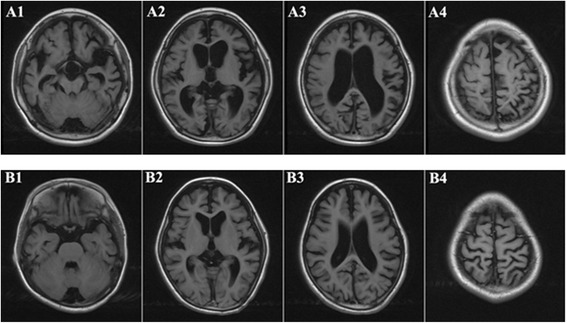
Brain MRI of the two prodbands (A: brother 10 years old; B: sister 9 years old): Axial T1-weighted images showed that the hemisphere sulci and schizencephaly general broadened and deepened. Double lateral and the third ventricle, cisterns were significantly dilated. The gray matter and white matter of the two hemisphere decreased. All of the above changes indicated ventricular dilatation and cortical atrophy in the two probands.
